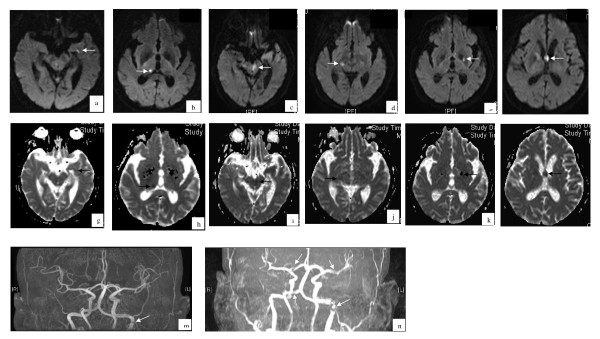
Brain magnetic resonance imaging (MRI) (diffusion-weighted images (1a-1f) and corresponding apparent diffusion coefficient maps (1h-1m)) and MR angiographic (1g and 1n) studies of Case 5. Figures 1a, 1b, 1h, and 1i (captured at the time of admission) show acute/subacute infarctions at the right thalamus (arrow) and left temporal region (arrowhead). Figure 1g (captured at the time of admission) shows suspected stenosis of the left internal carotid artery (arrow). Figures 1c-1f and 1j-1m (captured on the 17^th ^day after admission) show new infarctions in the midbrain (arrow), bilateral basal ganglia (arrowhead), and corpus callosum (arrows). Figure 1n (captured on the 17^th ^day after admission) shows multiple cerebral arterial stenoses (arrow).
